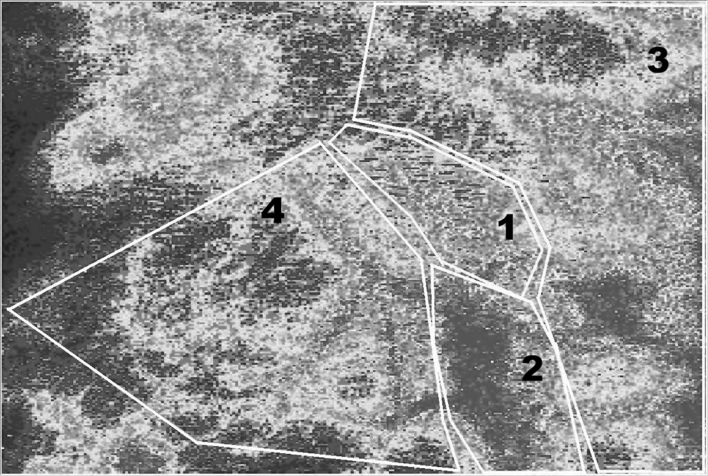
Laser Doppler scan with four regions of interest identified: *1* femoral artery, *2* distal femoral artery, *3* cranial region overlying the fracture zone and *4* caudal region
